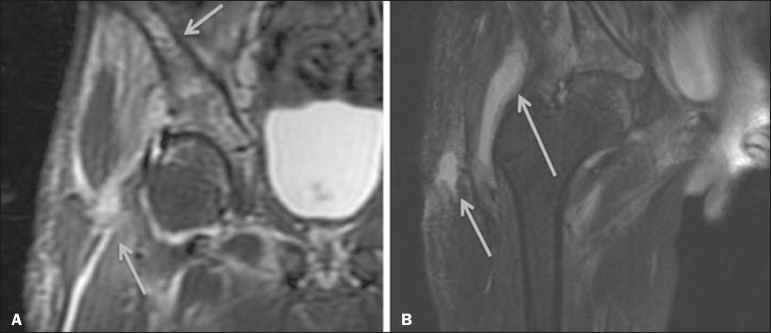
Pyomyositis in a 12-year-old male patient. Coronal fat-saturated T2-weighted MRI sequence showing joint effusion, osteitis, and edema involving the muscle-adipose planes of the gluteal region and root of the right thigh (A), featuring a voluminous, heterogeneous liquid collection, containing small foci of low signal intensity, likely of an infectious/inflammatory nature, located between the ventral portion of the gluteus medius muscle and that of the gluteus maximus muscle (B).
